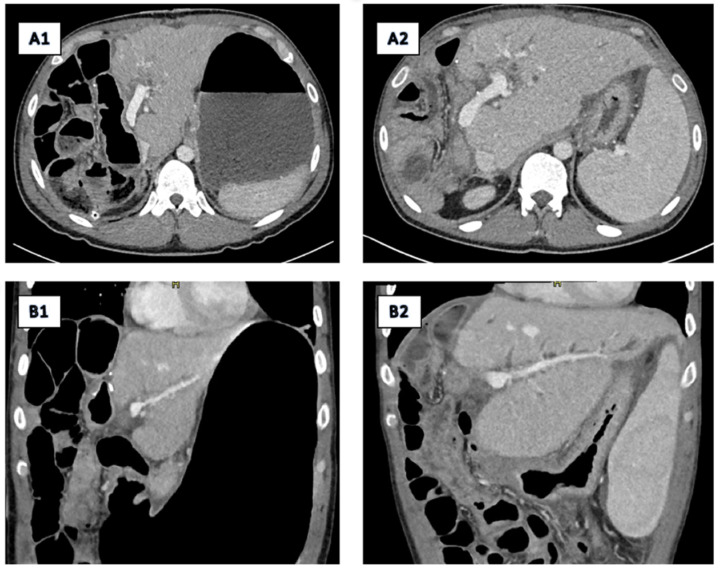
Postoperative CT scans obtained immediately after extended right hepatectomy (A1,B1) and at six-month follow-up (A2,B2) demonstrate hypertrophy of the liver remnant. Despite this compensatory regeneration, the residual liver volume remained insufficient to meet metabolic demands. The patient developed liver failure progressing to multiorgan dysfunction, consistent with small-for-size syndrome—a serious postoperative complication arising from an inadequate functional liver remnant following major hepatic resection.
